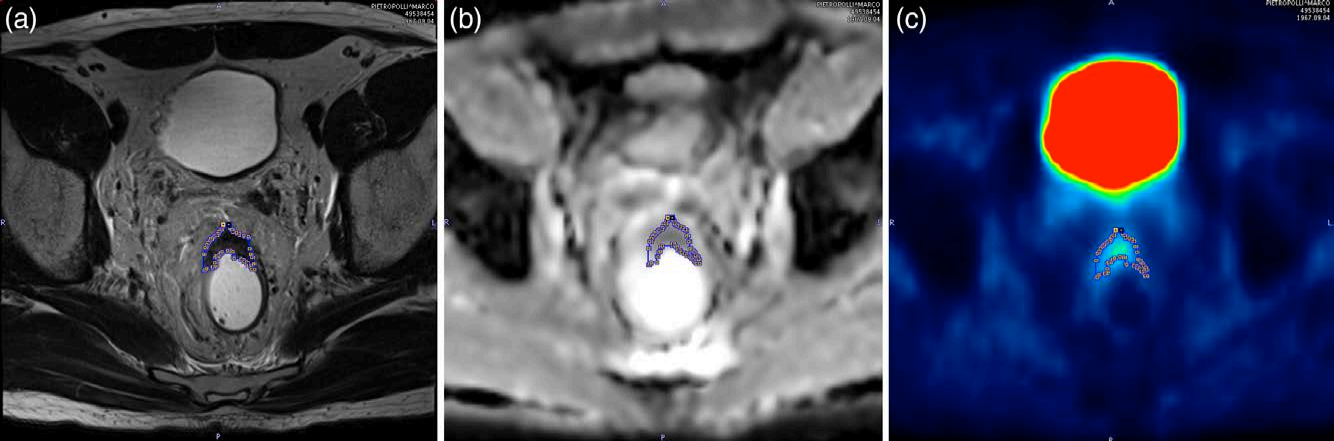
Manual region of interest (ROI) delineation with PMOD software, drawn along the boundaries of the rectal tumor in T2-weighted image (a) and then copied to the corresponding ADC map (b) and PET image (c).
